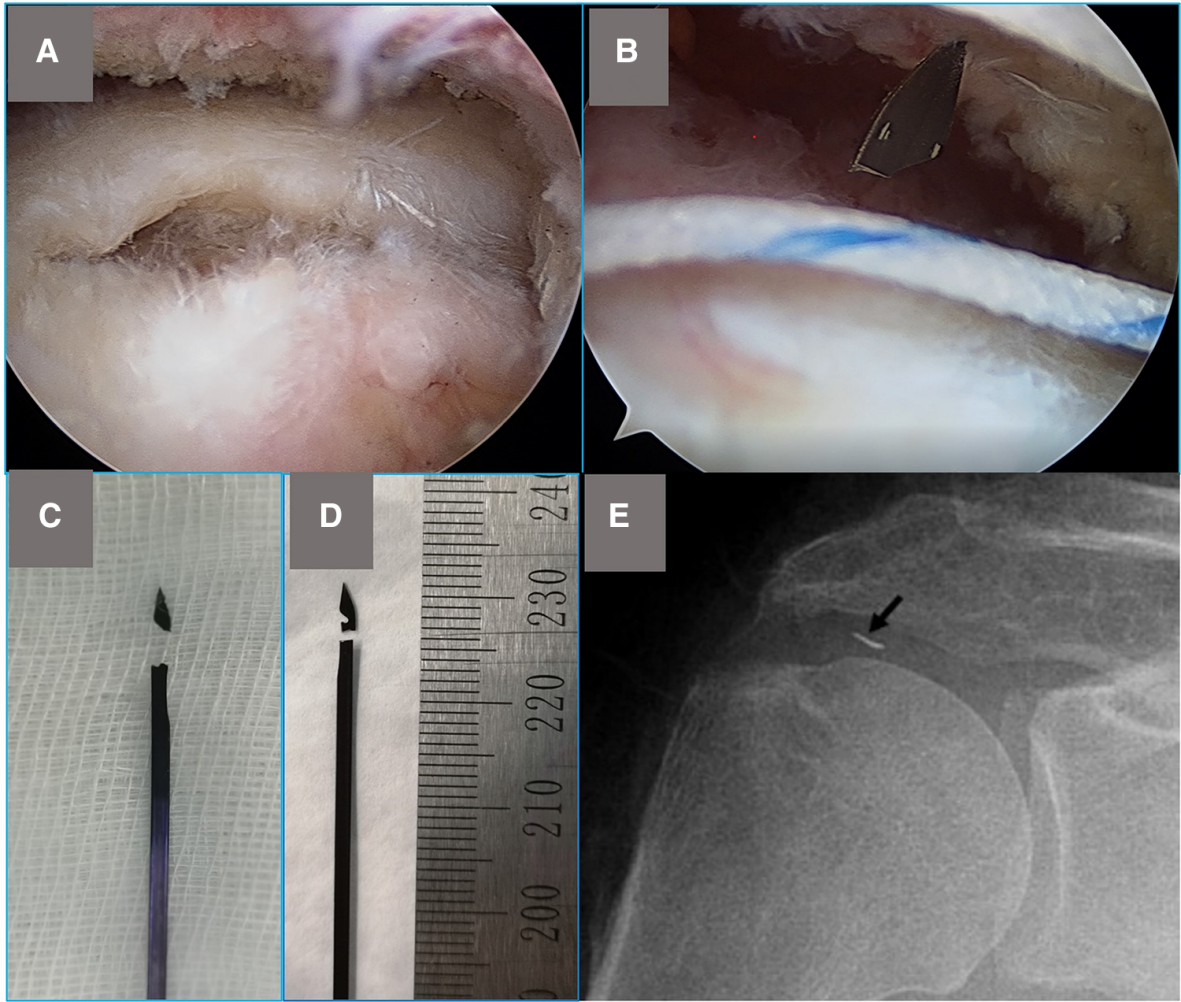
(A) Female, 59 years old, a full-thickness tear of the supraspinatus tendon was observed from the posterolateral approach. (B) The suture needle tip was broken during the operation, and the broken needle tip was found in the subacromial space. (C) Photograph of the broken needle of the TruePass™ suture passer, used in surgery (B). (D) Photograph of a needle of the TruePass™ suture passer snapped in advance. The break is located at the indentation in the needle. (E) Postoperative anteroposterior radiograph of a woman, 72 years old, 1 week after arthroscopic rotator cuff repair. The arrow points to a metallic foreign body that represents the broken tip of a suture passing device.
